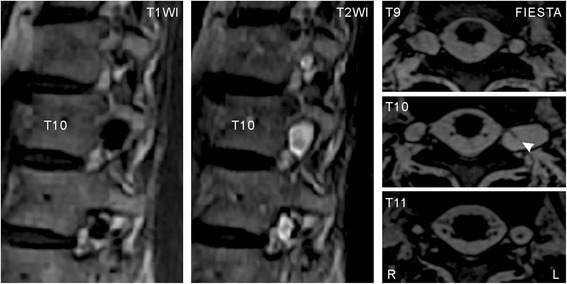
Magnetic resonance images at T9–T11 showing multiple perineural cysts. Sagittal views at the level of the left intervertebral foramina on T1- (*left*) and T2-weighted (*middle*) images show multiple cysts on the left side. In axial views acquired by fast imaging employing steady-state acquisition (FIESTA, *right*), a linear shadow (arrowhead) is seen in the largest cyst at left T10
